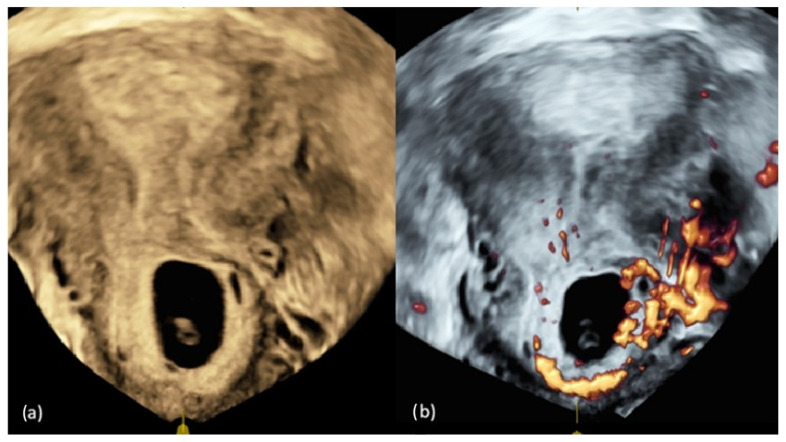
(a) Three-dimensional transvaginal ultrasound image obtained at the initial diagnostic stage in a patient with a viable cervical pregnancy at approximately 6 weeks of gestation. The coronal plane shows the gestational sac implanted within the cervical canal; (b) Power Doppler ultrasound of the same view demonstrating marked peritrophoblastic vascularization. Doppler imaging was performed to assess the risk of hemorrhage associated with the abnormal implantation site.
